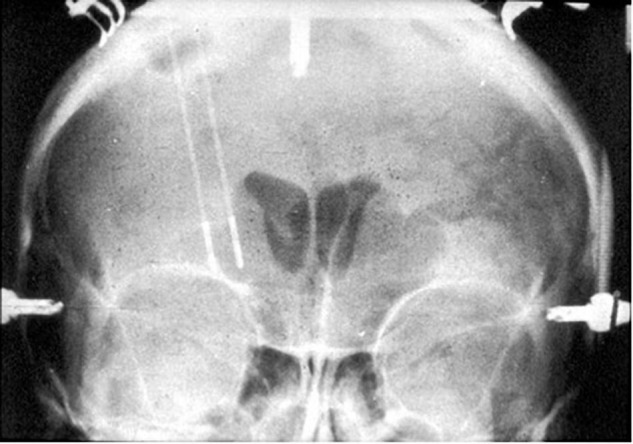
Coronal X-ray of electrodes in place for creation of Leksell’s right frontal capsulotomy lesions.
